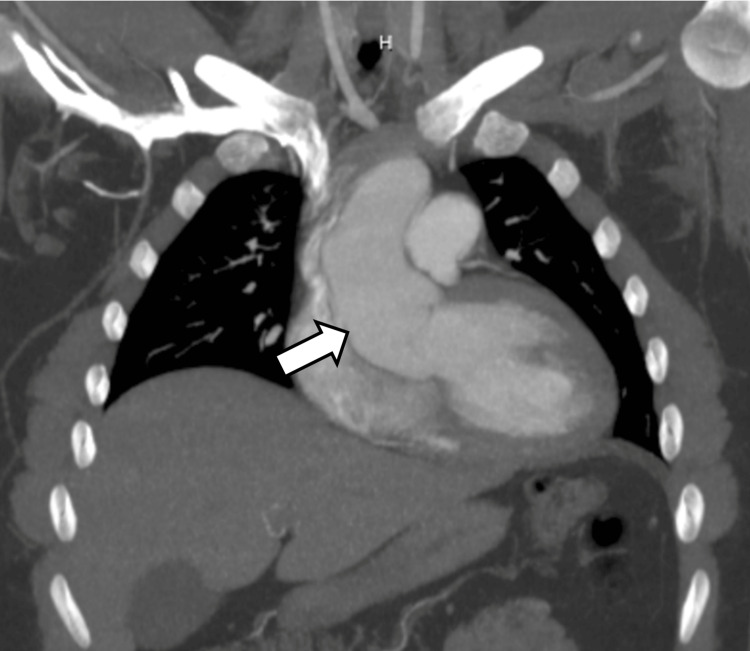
CT angiogram chest with contrast revealing a transverse diameter of the ascending aorta at the level of the aortic root at 4.6 cm
